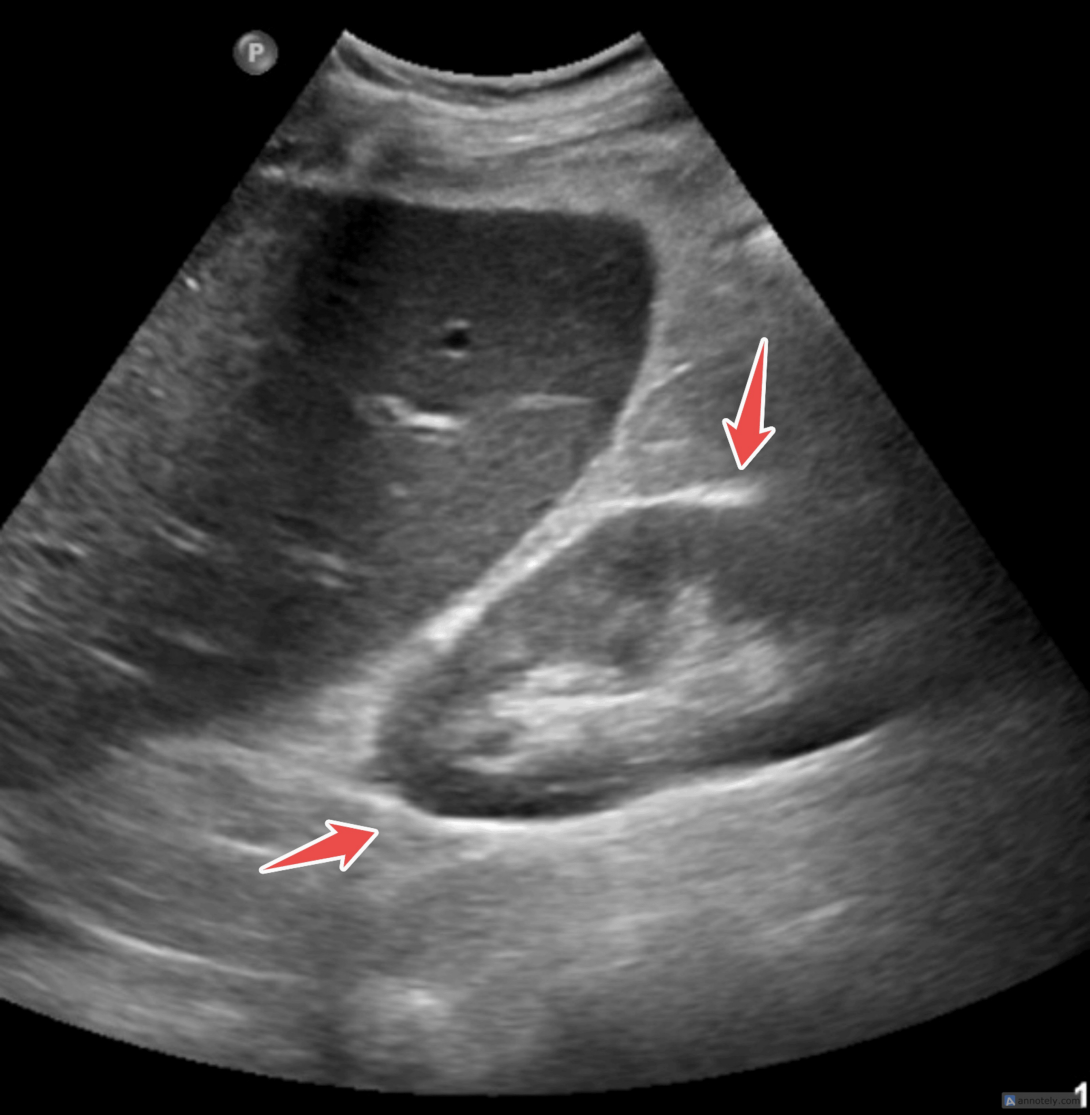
Abdominal ultrasound showing sign of cholecystitis.
